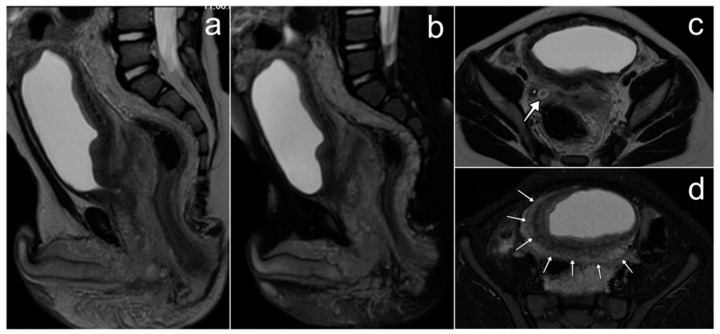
The pelvic MRI in Case 3 (a–d). A thick arrow in (c) marks the largest lesion forming plexiform neurofibroma with a typical “target sign”. Short arrows in (d) indicate the thickened, infiltrated urinary bladder wall. (a,c)—FSE/T2; (b,d)—STIR.
